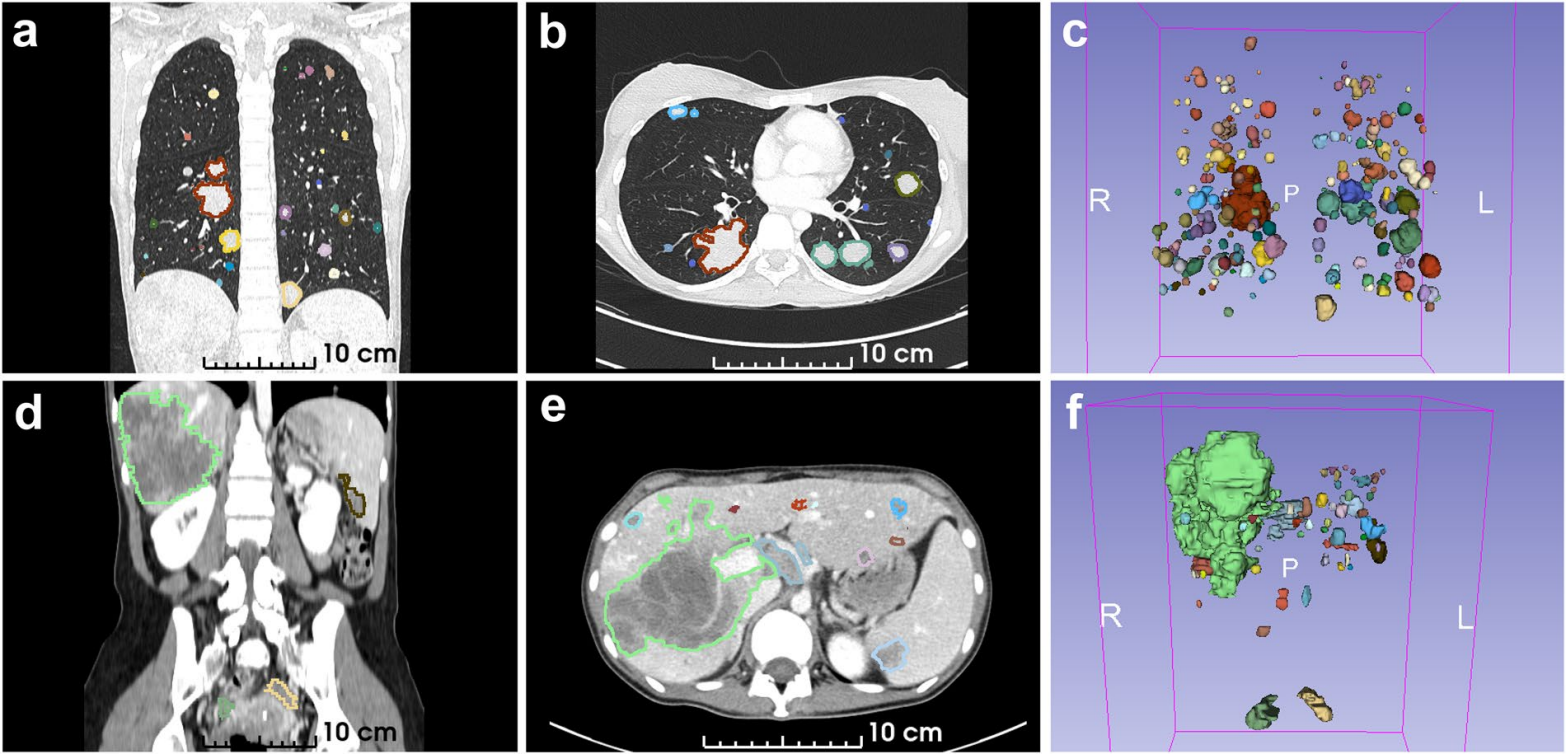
Examples of annotated lesions in CT images. Each row corresponds to a series obtained from the same patient and CT study. (a–c) Thorax. (d–f) Abdomen. Each column shows a specific view of the CT image, displaying annotated lesions in color. From left to right: coronal plane, axial plane, and 3 d rendering.
